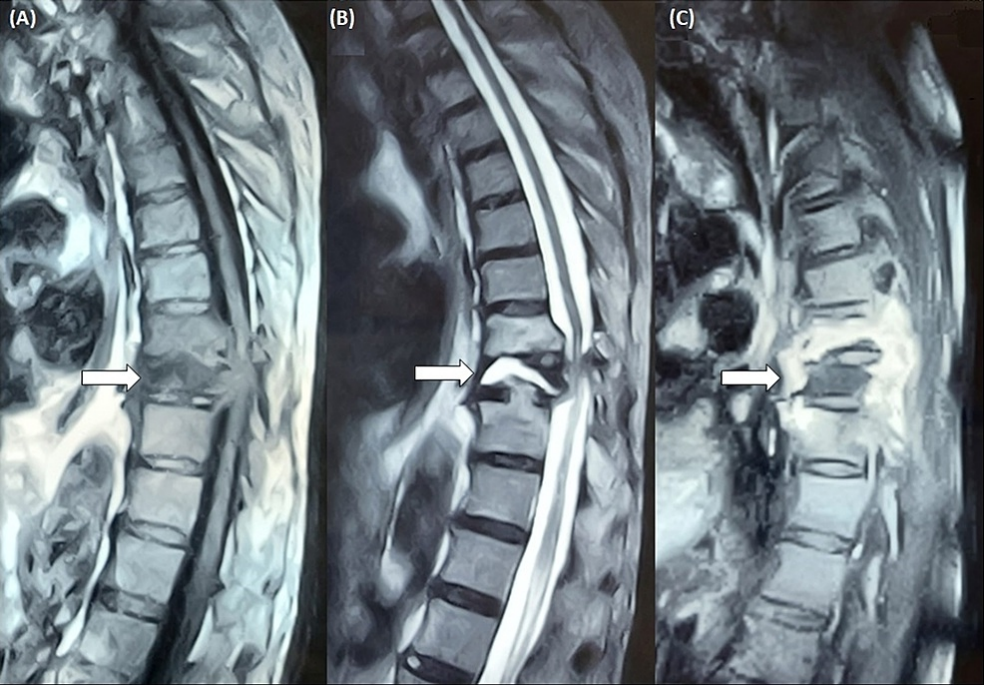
Magnetic resonance imaging of the thoracic spine (sagittal plane) (A) T1-weighted image shows a hypointensive lesion at the level of the ninth thoracic vertebra with protrusion to the spinal cavity and prevertebral space (arrow). There is collapse and sclerosis of the T9 vertebral body. (B) T2-weighted image shows hyperintensity of the same dorsal vertebra (arrow) and spinal compression. (C) T1-weighted image post-gadolinium highlights the extension of the lesion to the prevertebral planes, as well as T8 and T10 (arrow).
